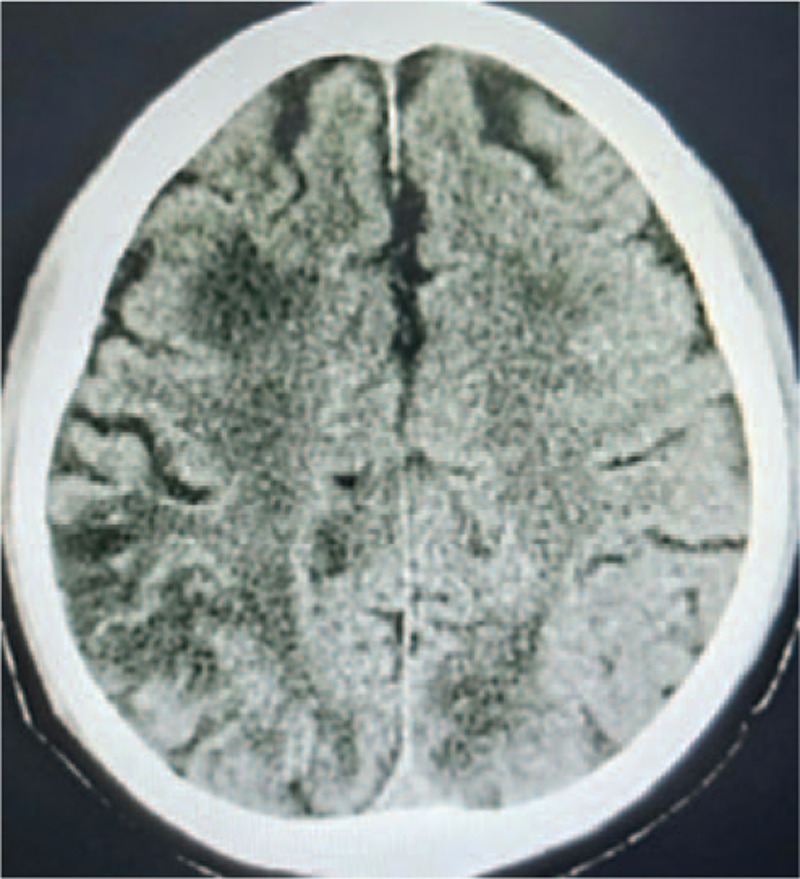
Brain computed tomography (CT) image on August 1, 2018.
